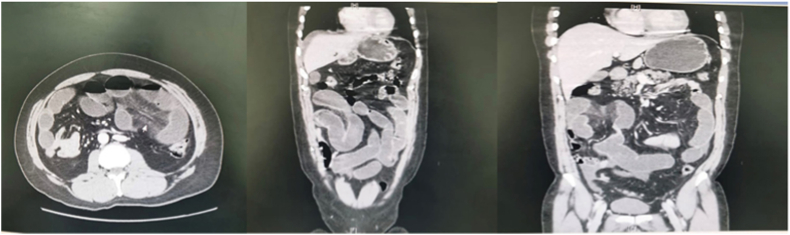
Computed tomography of the abdomen showing high-grade intestinal obstruction with a transition zone.
